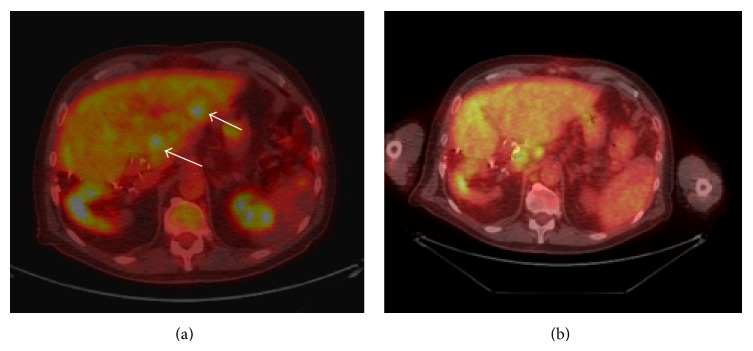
Axial PET-CT images (a) before biliary drainage and SIRT and (b) two months after SIRT, at the level of segments 2 and 3. Significant response to treatment is evident, with resolution of FDG avid hepatic metastases (arrows). However, progression of extrahepatic disease was noted in portocaval and periaortic nodes.
